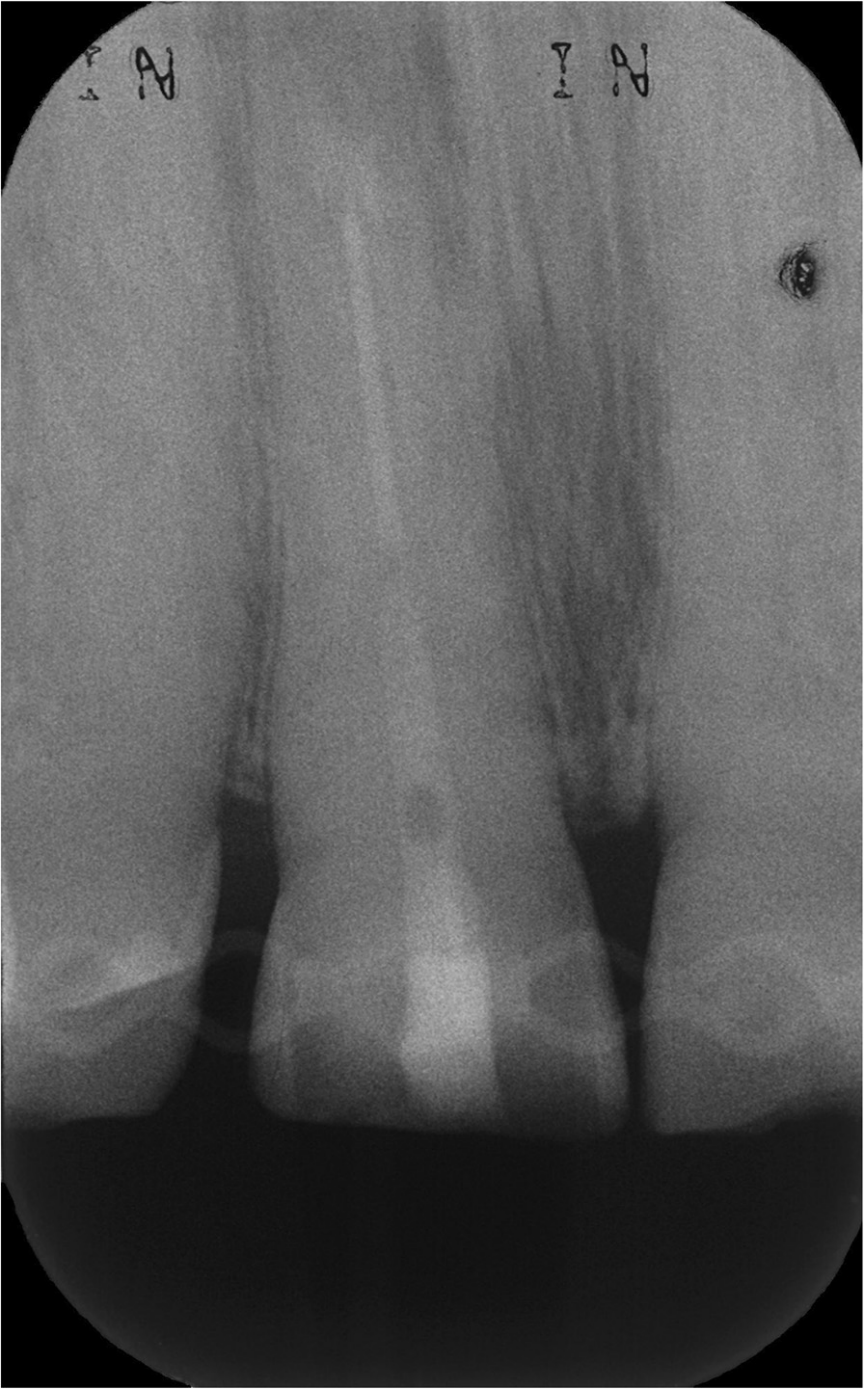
Control radiograph of tooth 11 after replantation.
